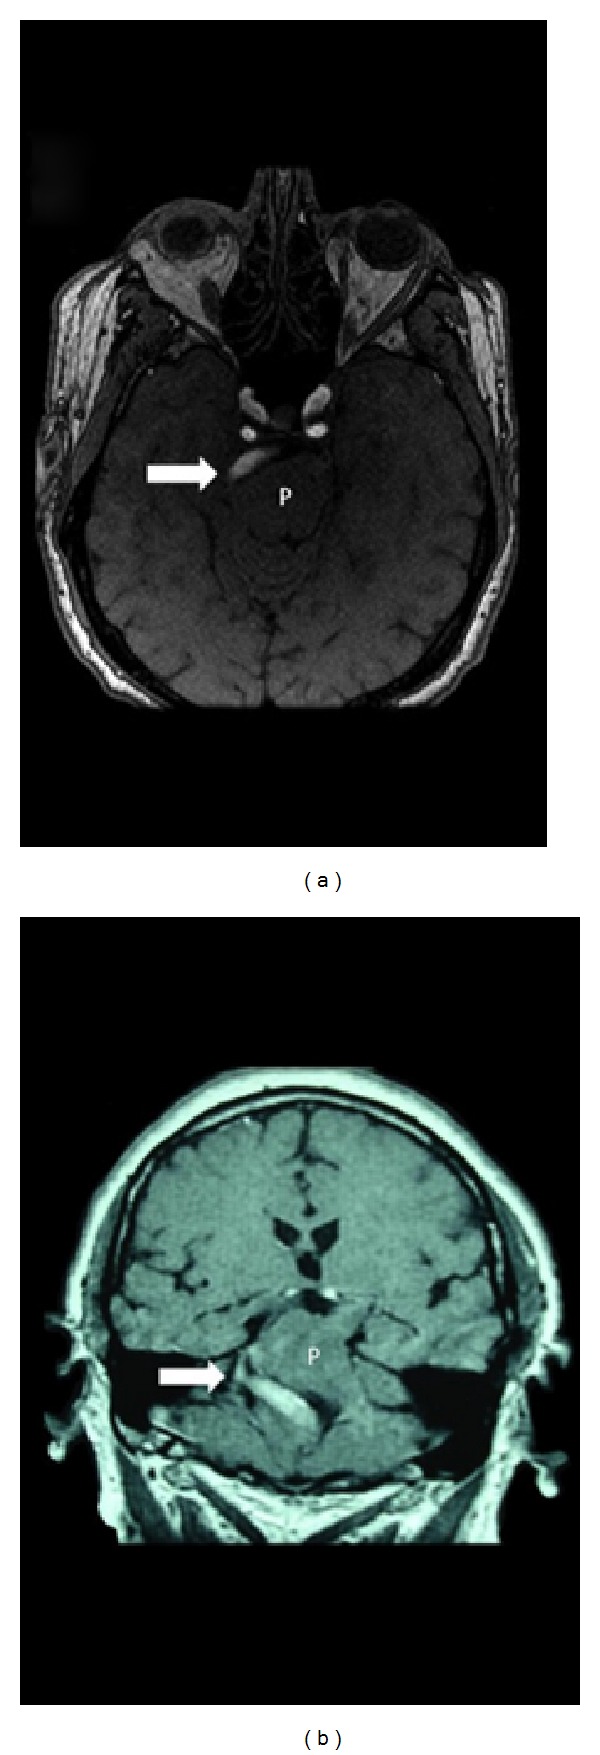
Neurovascular conflict: (a) (axial) and (b) (coronal) MRI reconstruction from a 3D-FSPGR study shows a dolichoectatic basilar artery (arrow) crossing and displacing upper pons (P) with compression of the right trigeminal nerve at the root entry zone.
